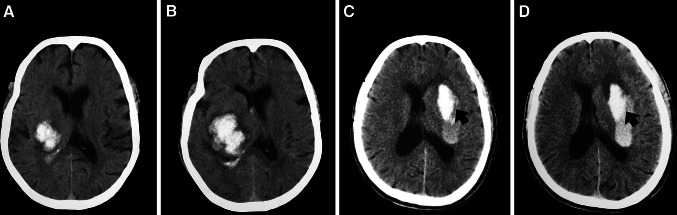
Haematoma heterogeneity and haematoma growth border. These two patients were admitted to our hospital with the impression of spontaneous ICH. Patient 1: a CT on admission shows haematoma heterogeneity with an initial haematoma volume of 15 mL. b Repeated CT after 32 h shows a haematoma volume of 30 mL. Patient 2: c CT on admission shows haematoma growth border (black arrow). d Repeated CT after 10 h shows haematoma expansion with increased midline shift
